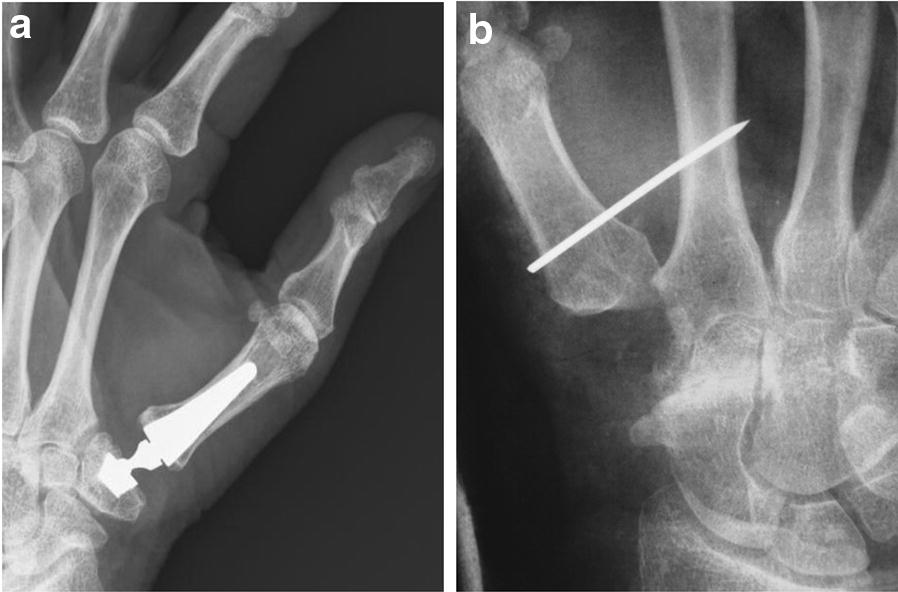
X-ray images after Ivory prosthesis implantation (a) and resection arthroplasty (b)
